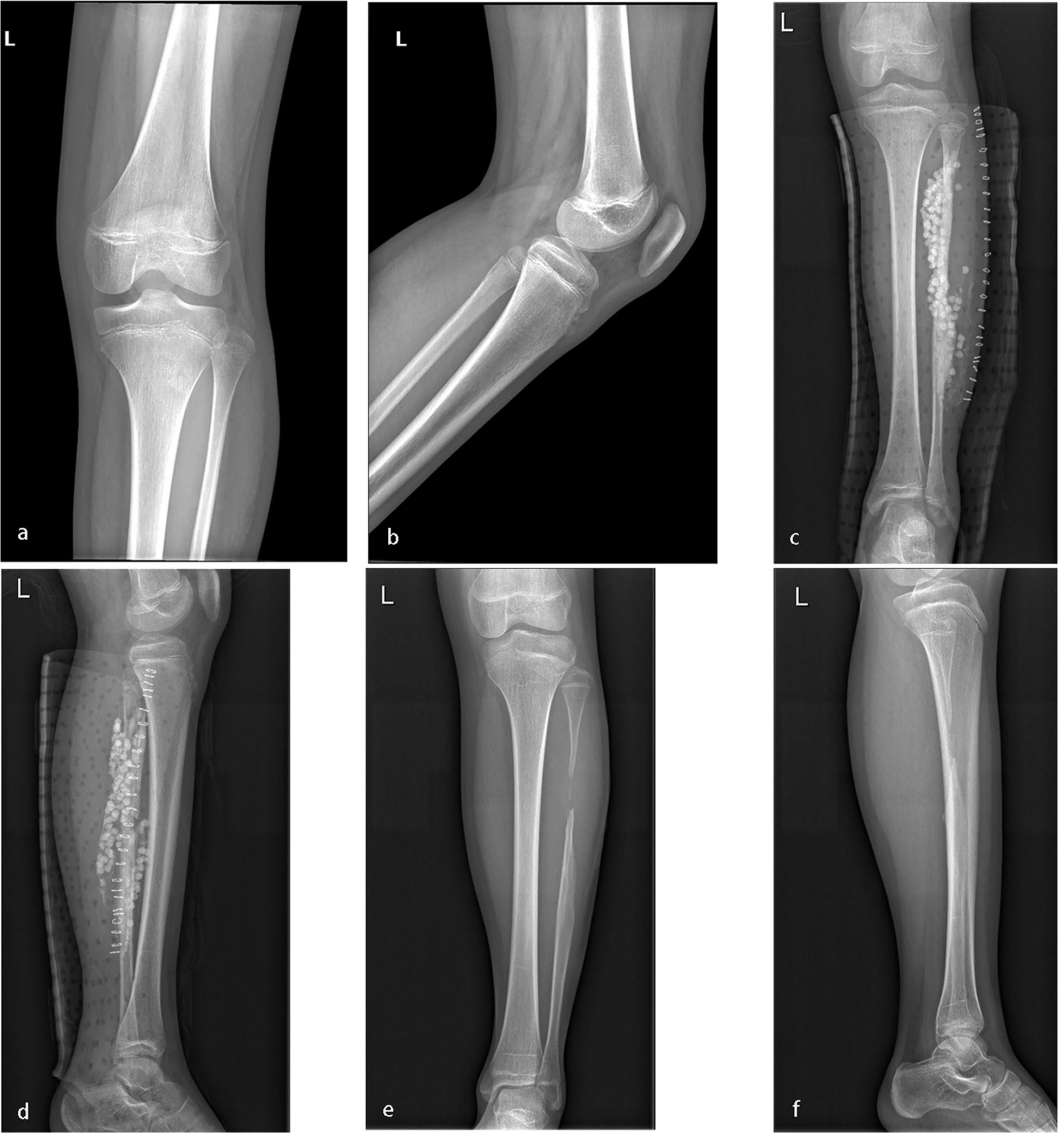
a-b: A ten-year-old girl suffered left proximal fibular HO. c-d: Vancomycin impregnated CS was used to fill the void left by decompression and debridement. e–f: X-ray examination indicated osteolysis 18 months postoperatively
